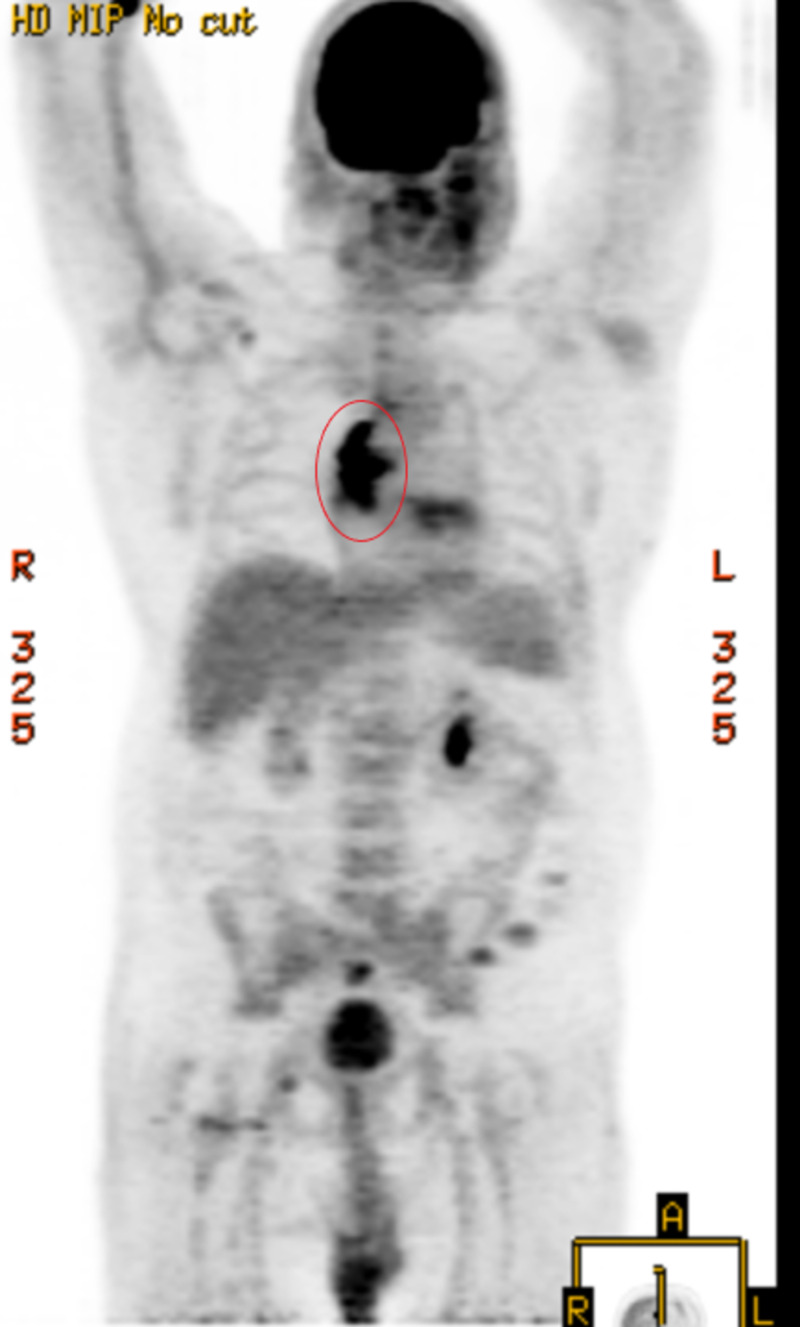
F-18-Fluorodeoxyglucose Positron Emission Tomography Scan A diagnostic FDG PET scan found moderate to marked hypermetabolism within the sternotomy line with maximum standard uptake value (SUV) 4.6. FDG PET: F-18-fluorodeoxyglucose positron emission tomography
